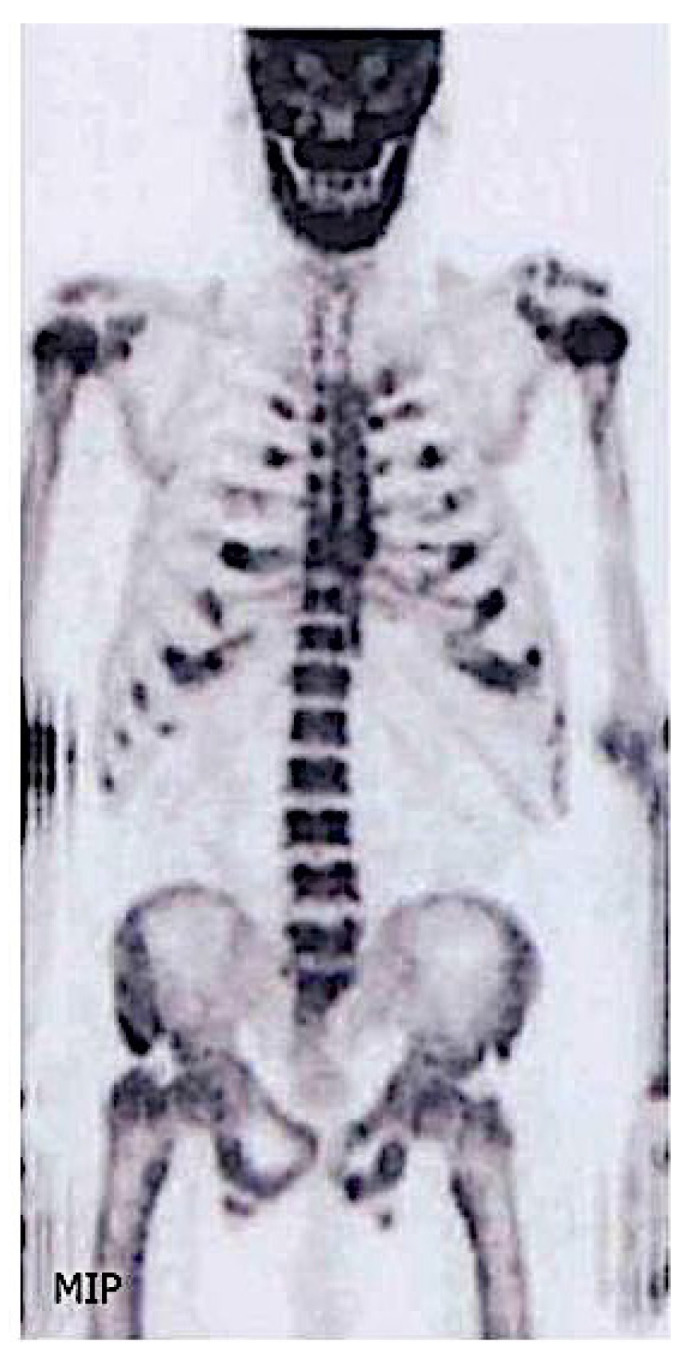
Bone scintigraphy: skeletal uptake of ^99m^Tc-labelled diphosphonate in a patient with hyperparathyroidism. There is increased bone turnover shown by increased symmetric tracer uptake throughout the skeleton, including calvaria, mandible, humeral head, accentuating the contrast between bone and soft tissue, and a beading pattern at the costochondral junctions.
